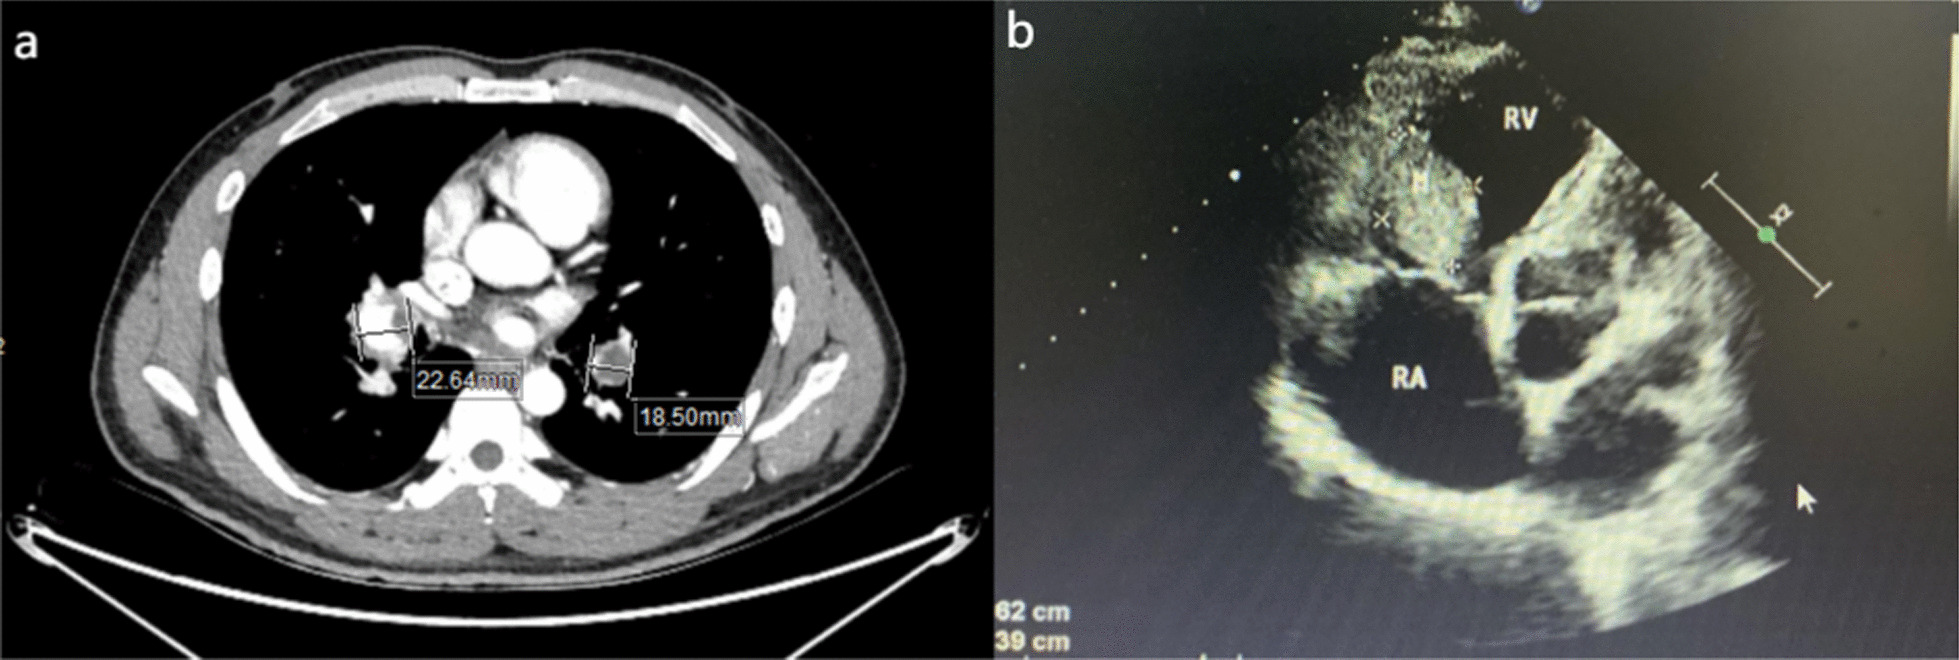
a Contrast-enhanced computed tomography showed emboli formation in the pulmonary artery and its branches; b Transthoracic echocardiography before surgery showed a right ventricular space-occupying lesion
